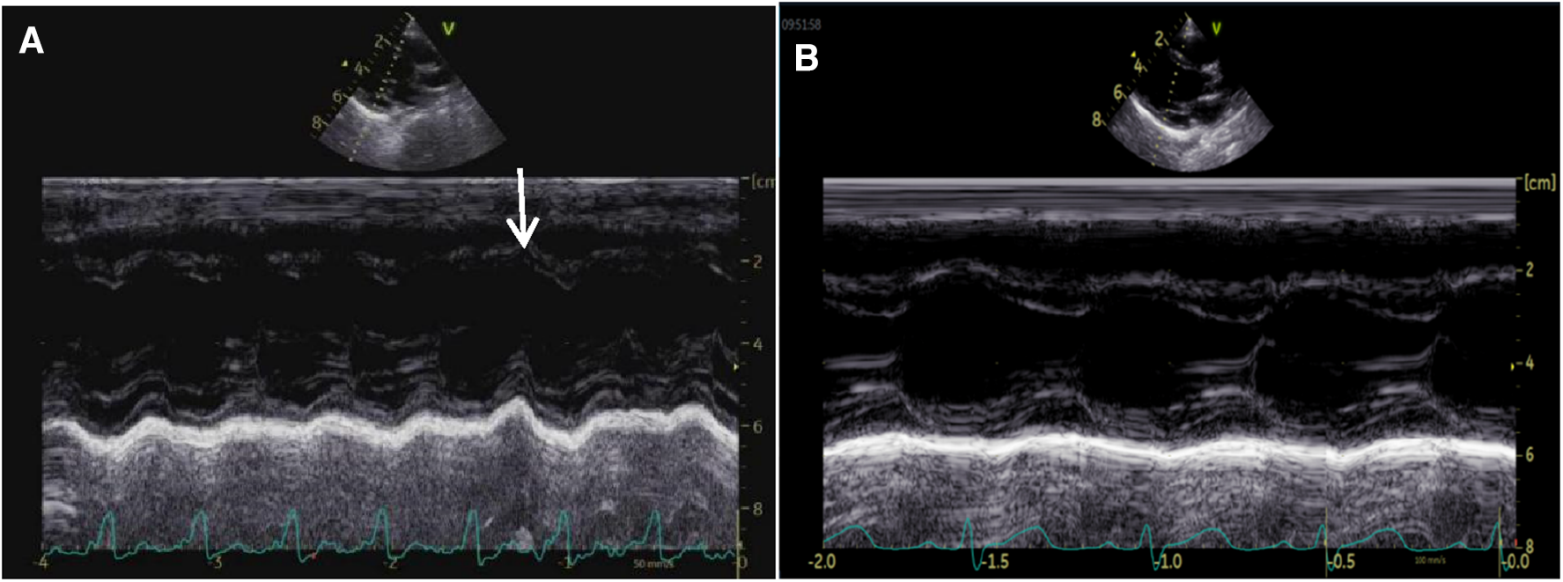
M-mode tracing from a parasternal long-axis view of case 2 before the therapy of amiodarone, showing interventricular septal paradoxical motion (A, arrow). The ventricular septum and LV post wall moved normally 6 months after the therapy of amiodarone (B).
